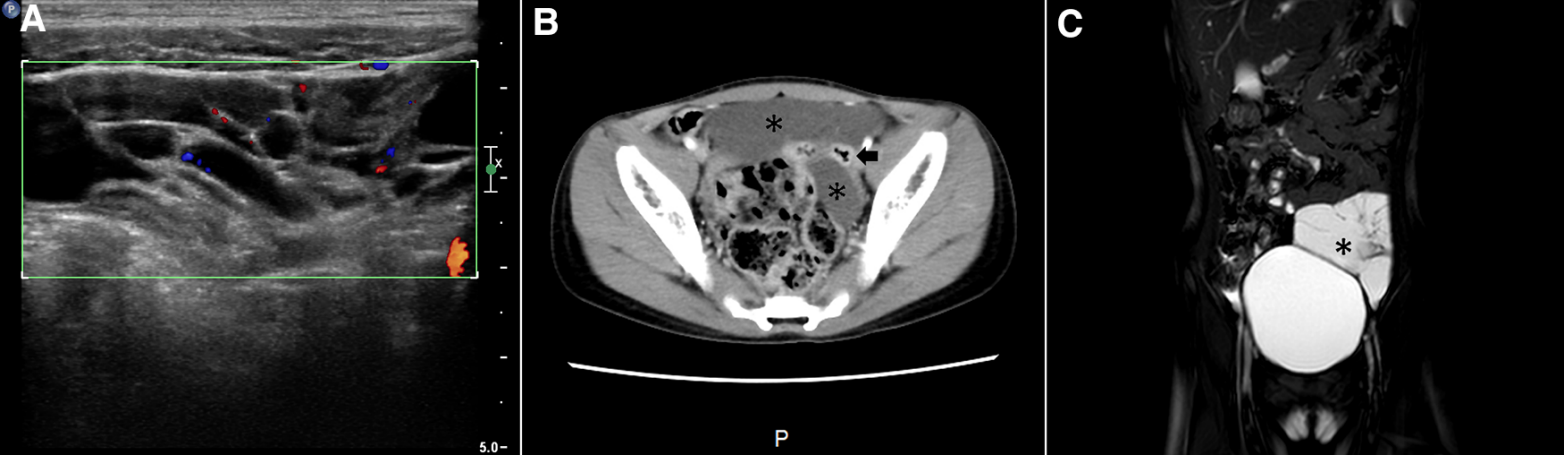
Imaging examination of a patient with a dumbbell-type ileal mesenteric cyst. (A) Sonography demonstrated a hypoechoic cyst with multiple internal septations; (B) enhanced CT scan showing a cyst (asterisk) located on both sides of the intestine (arrow), without contrast enhancement; (C) MRI showing an irregular cyst (asterisk) with a sharp margin and septation on a T2-weighted image.
